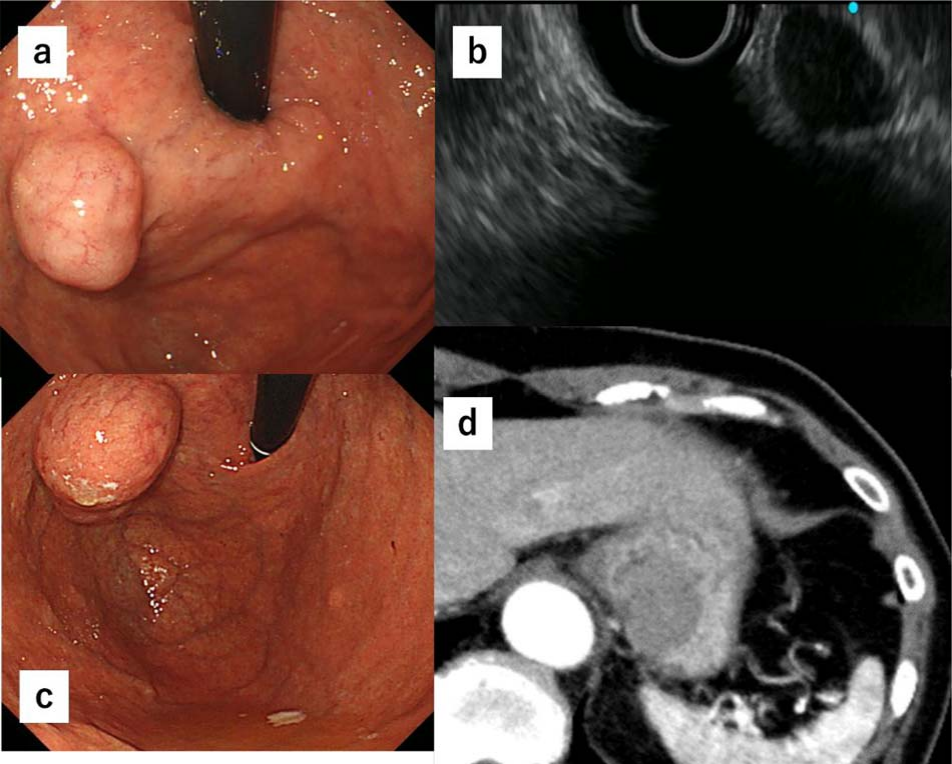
(a) A submucosal tumor measuring ∼2 cm is found at the posterior wall of the upper body of the gastric body near the EGJ by upper endoscopy. (b) Endoscopic ultrasonography shows a gastric hypoechoic tumor from the fourth layer suggesting GIST. (c) The tumor shows increase in size by ∼1 cm compared with 1 year ago. (d) CT reveals an intraluminal growth type submucosal tumor measuring 3.5 cm in diameter and no metastasis.
